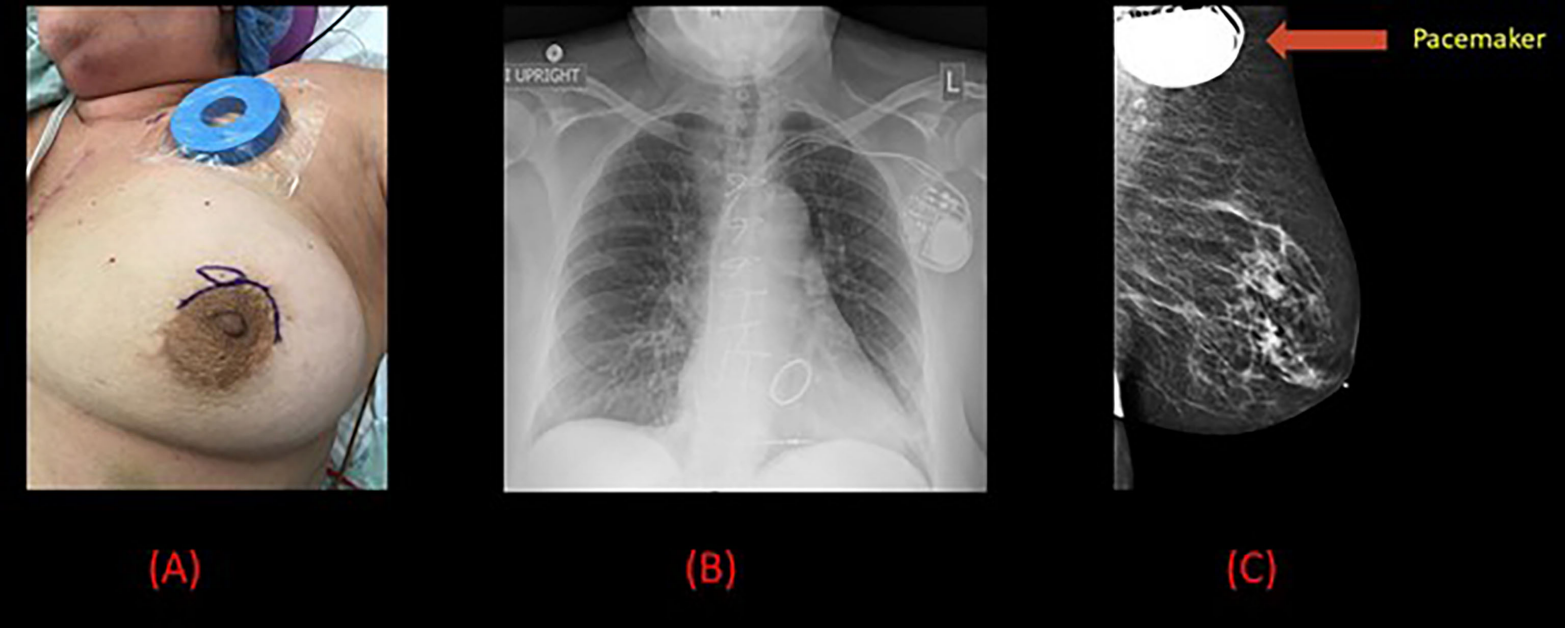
Pre-operative and radiological images for patient 2. (A) Pre-operative image of the left breast with a magnet placed on the pacemaker and surgical marking indicating the tumor site. (B) Chest x-ray demonstrating left sided pacemaker with leads projecting over the right atrium and right ventricle. (C) Mammogram demonstrating a 7 mm spiculated mass in the left breast along with the pacemaker.
